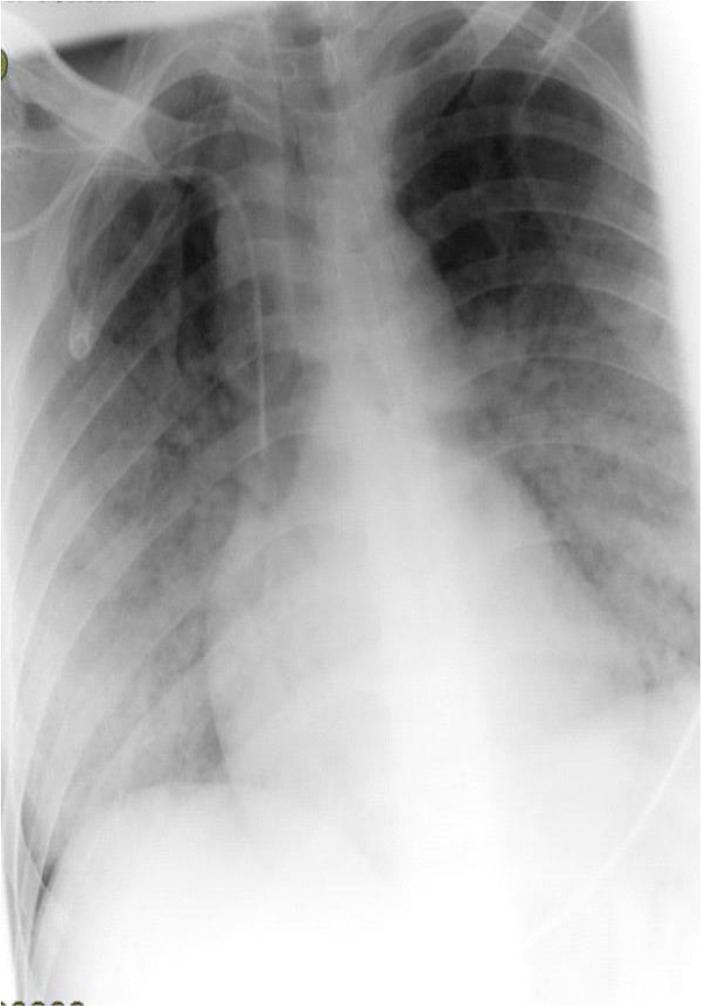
Chest X-Ray showed diffuse opacities
